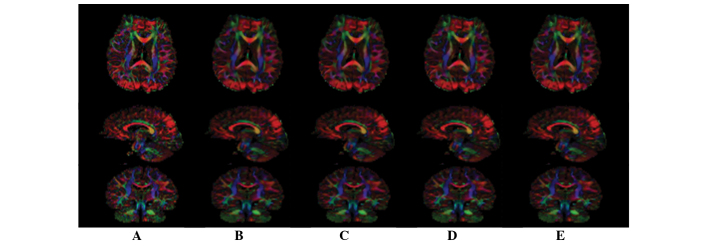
In vivo* PD maps prior to and following denoising. PD map of (A) original image, and denoising with (B) RD, (C) LED and (D) ED weighting and (E) UNLM in DWI space. Upper row, axial plane; middle row, sagittal plane; lower row, coronal plane; PD, principal direction; RD, Riemannian distance; LED, Log-Euclidean distance; ED, Euclidean distance; UNLM, unbiased non-local mean; DWI, diffusion-weighted image.
